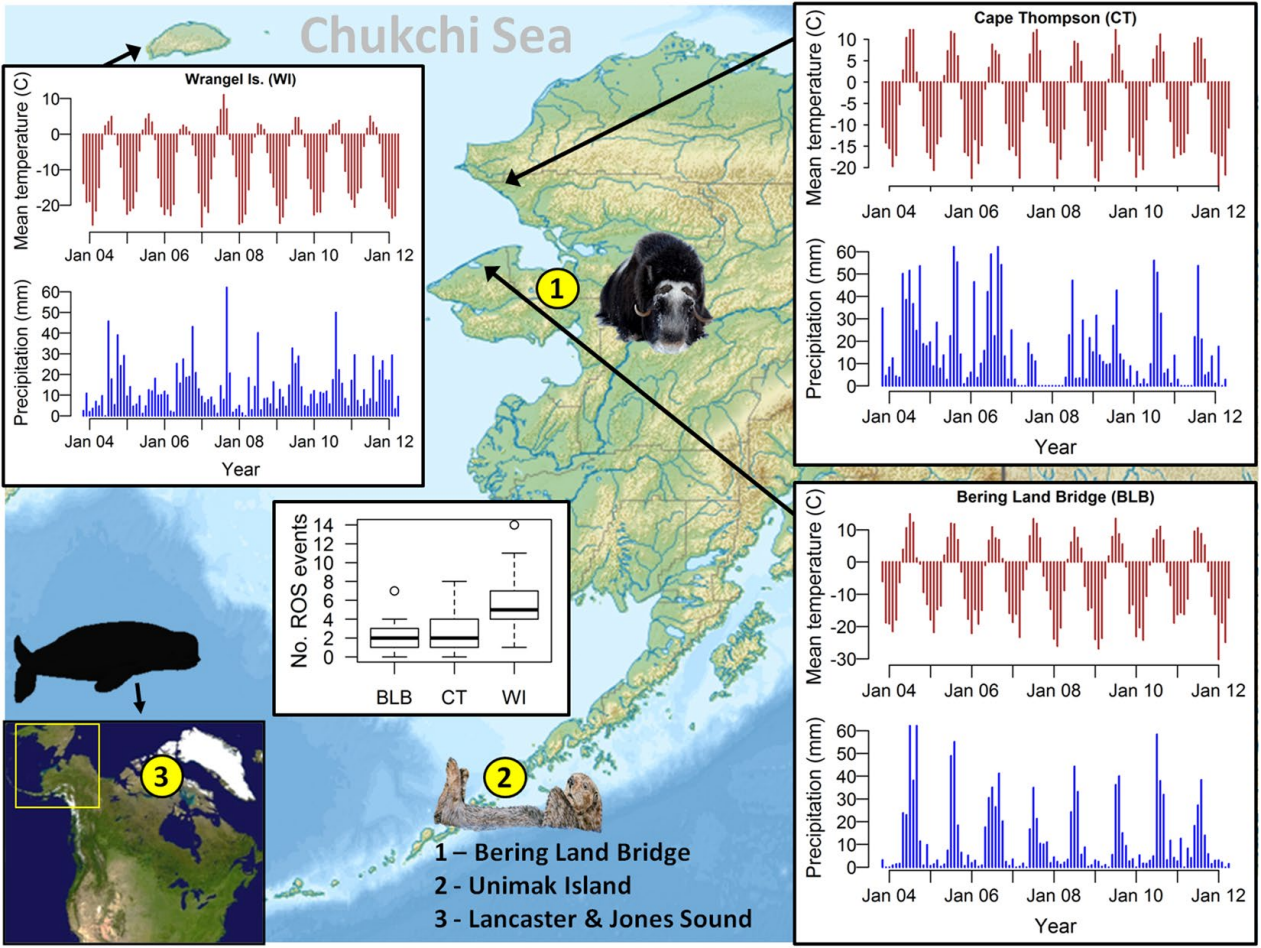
Monthly weather profiles (2004–2012) and number of ROS events/year (2006–2012) for our three study sites: Bering land Bridge (BLB), Cape Thompson (CT), and Wrangel Island (WI). Yellow circles and figurines denote locations and species/taxa affected by extreme icing events discussed in main text. Map of Beringia was modified by the authors from the original by Nikita A. Zemin (https://en.wikipedia.org/wiki/Juneau,_Alaska#/media/File:Relief_map_of_USA_Alaska.png), CC-BY SA 3.0 (https://creativecommons.org/licenses/by-sa/3.0/).
